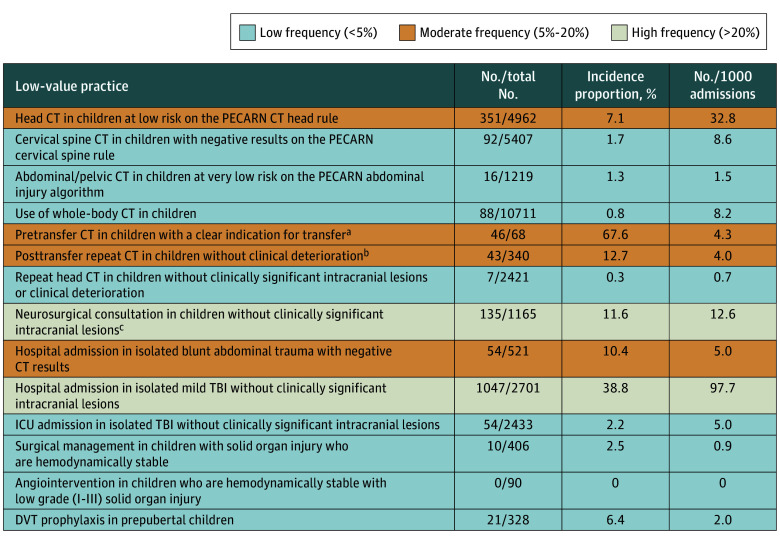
Relative (Incidence Proportions) and Absolute (Case Volumes) Frequencies of Low-Value Practices in Pediatric Trauma Admissions, 2016-2022 CT indicates computed tomography; DVT, deep vein thrombosis; ICU, intensive care unit; PECARN, Pediatric Emergency Care Applied Research Network; and TBI, traumatic brain injury. ^a^Applies to level III/IV referral centers. ^b^Applies to pediatric trauma centers and level I/II adult trauma centers. ^c^Consultation in the emergency department for neurosurgical centers (level I /II) and transfer to neurotrauma trauma centers for level III/IV centers.
